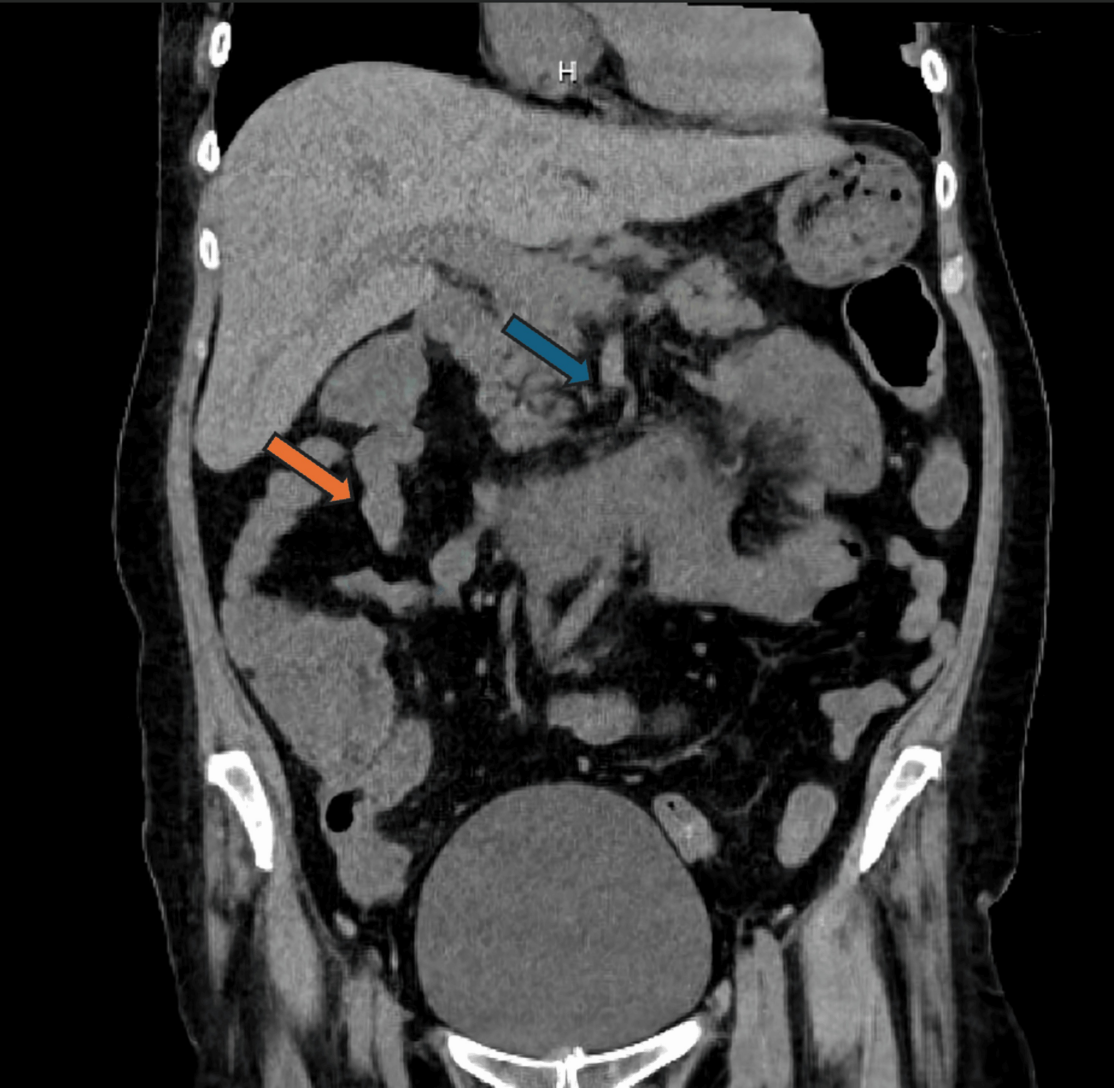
Coronal computed tomography scan of the abdomen; orange arrow represents the descending colon and blue arrow represents the transverse colon
